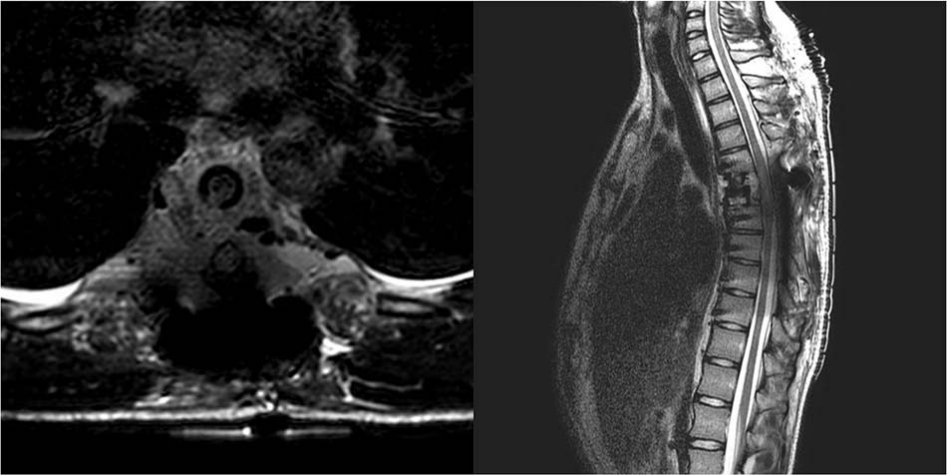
Immediate postoperative MRI showing no residual signs of thoracic spinal cord compression.
